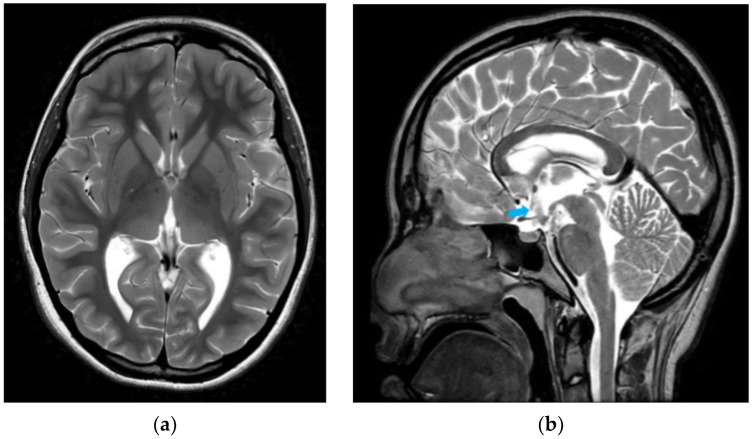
Representative T2-weighted sequence MRI brain images 6 months after ETV in axial (a) and sagittal (b) views, respectively. (a) In this MRI image in the axial direction, the ventricle size remains reduced in comparison to before surgery. (b) In the corresponding sagittal view of the same MRI study, there is radiological evidence of a flow void (blue arrow) into the prepontine cistern.
